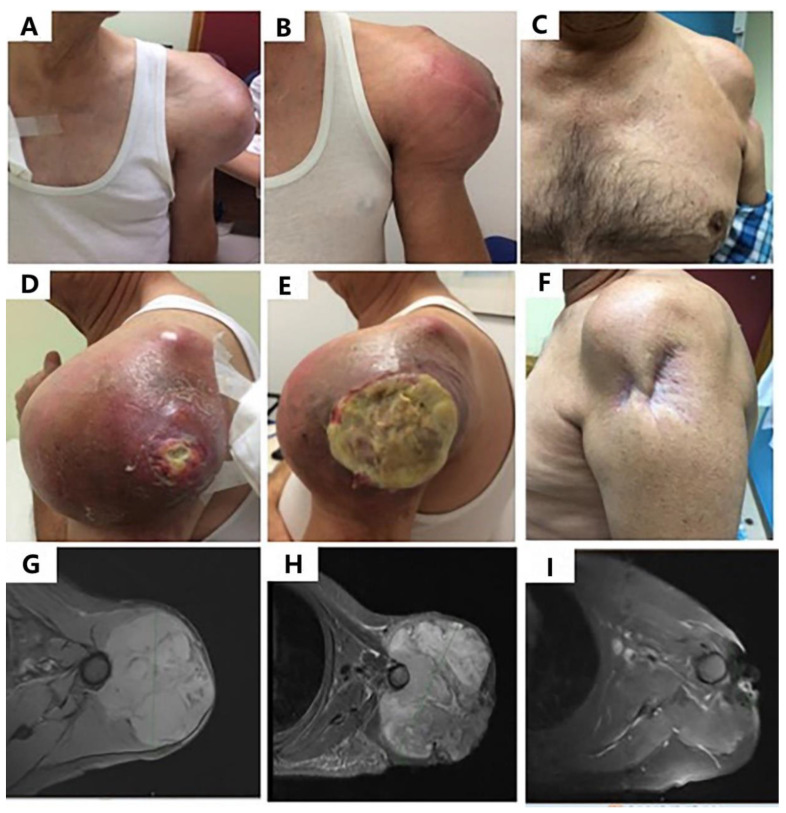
Clinical and RECIST partial response in a 59-year-old man with a metastatic leiomyosarcoma. The patient received trabectedin plus RT (30 Gy in 10 fractions) after rapid progression to doxorubicin-olaratumab. (A,D) Baseline study (November 2018); (B,E) Skin ulceration after Cycle 1 of trabectedin and RT (Dec 2018); (C,F) Long-lasting partial response (September 2019). (G–I) MRI images. (G) Baseline to previous line (September 2018); (H) Baseline to trabectedin-RT (November 2018); (I) Partial response (August 2019).
